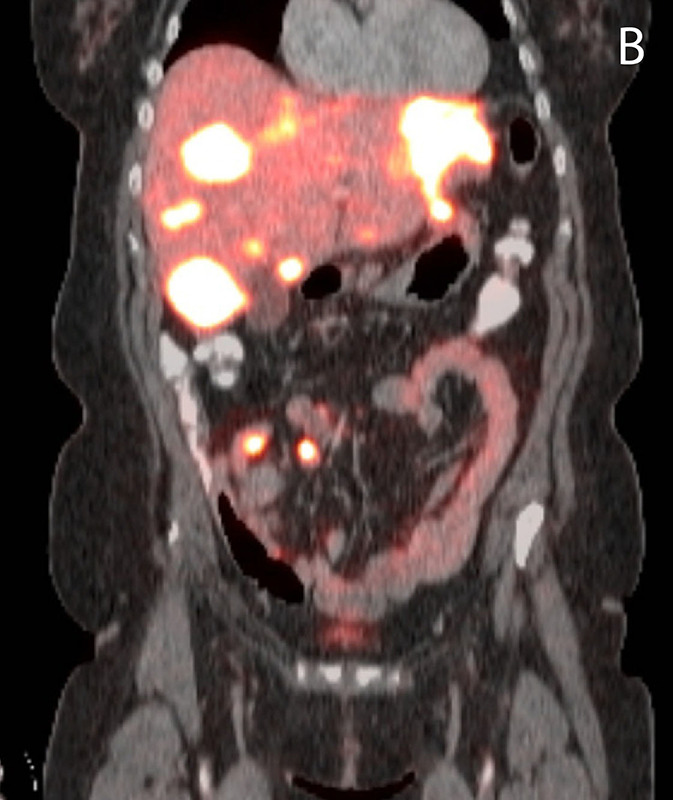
Primary small intestine NET with diffuse liver metastases: (A) coronal contrast‑enhanced CT shows a hypodense mesenterial mass (arrow) with central calcifications. (B) Al18F‑NOTA‑Octreotide PET‑CT shows multiple hyperenhancing liver nodules.
